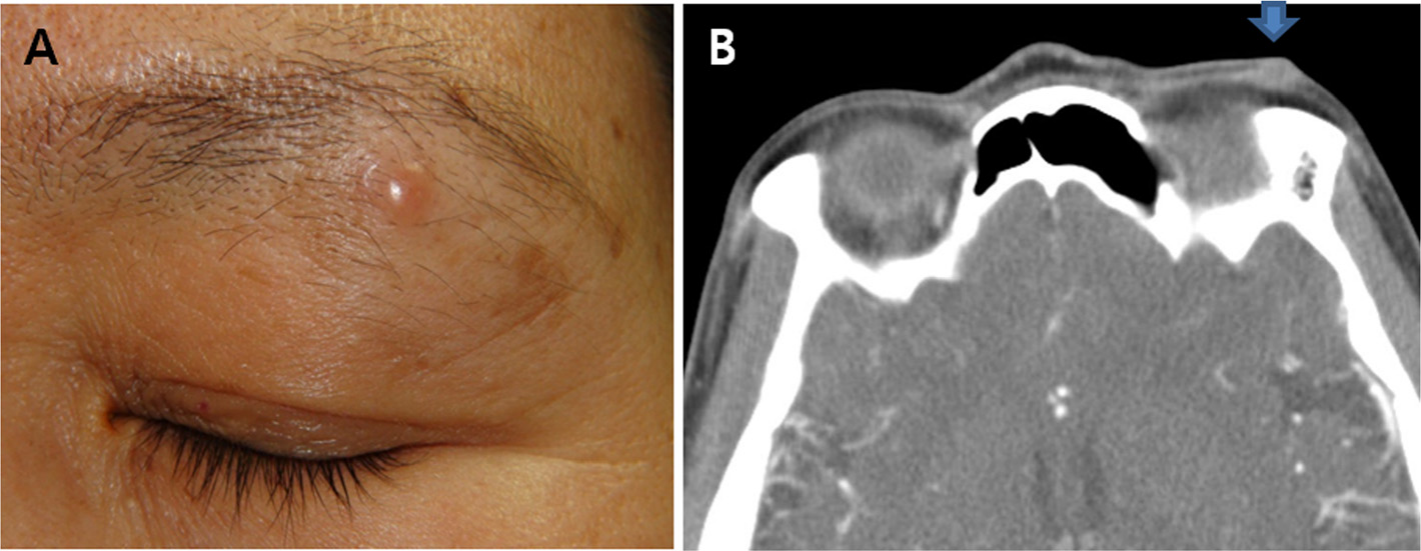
Clinical presentation. (A) Clinical photograph of a patient presenting with a 5.5 mm × 4.5 mm soft non-tender nodular mass with no overlying skin change. (B) An orbital CT scan showing a 7-mm nodular homogenous enhancing mass adherent to the skin of the left upper lid (arrow).
